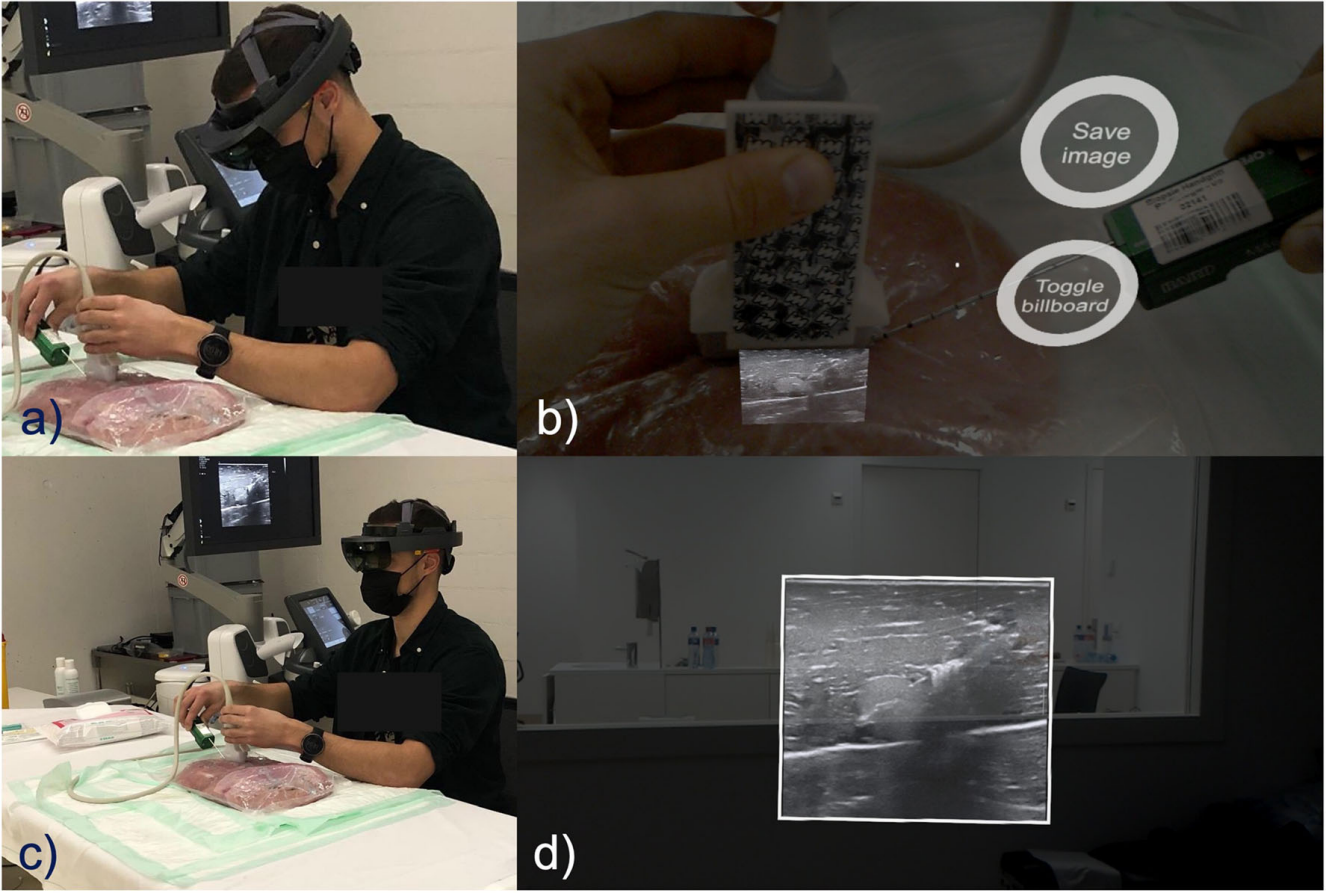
Ultrasound guided biopsy using augmented reality (AR US). a, b Using a QR code attached to a US transducer to track a US probe within the room, an in situ actual-sized image is projected directly below the US probe at the exact anatomical position in size real time. c, d Lifting the head initiates display of a second bigger image (same size as on an ultrasound screen) that follows the eyes of the operator
